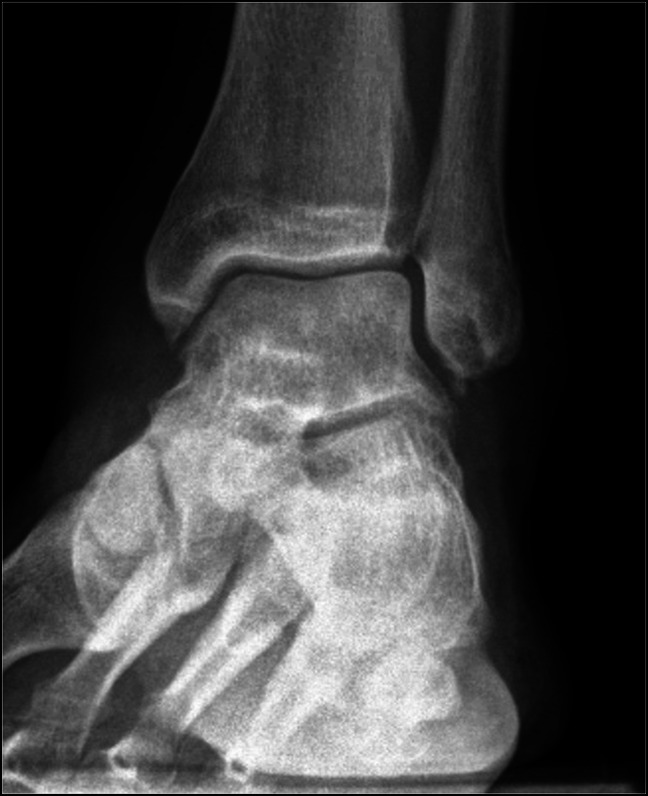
Ankle mortise weight-bearing radiograph image 6 months postreduction without signs of avascular necrosis of the talus.
